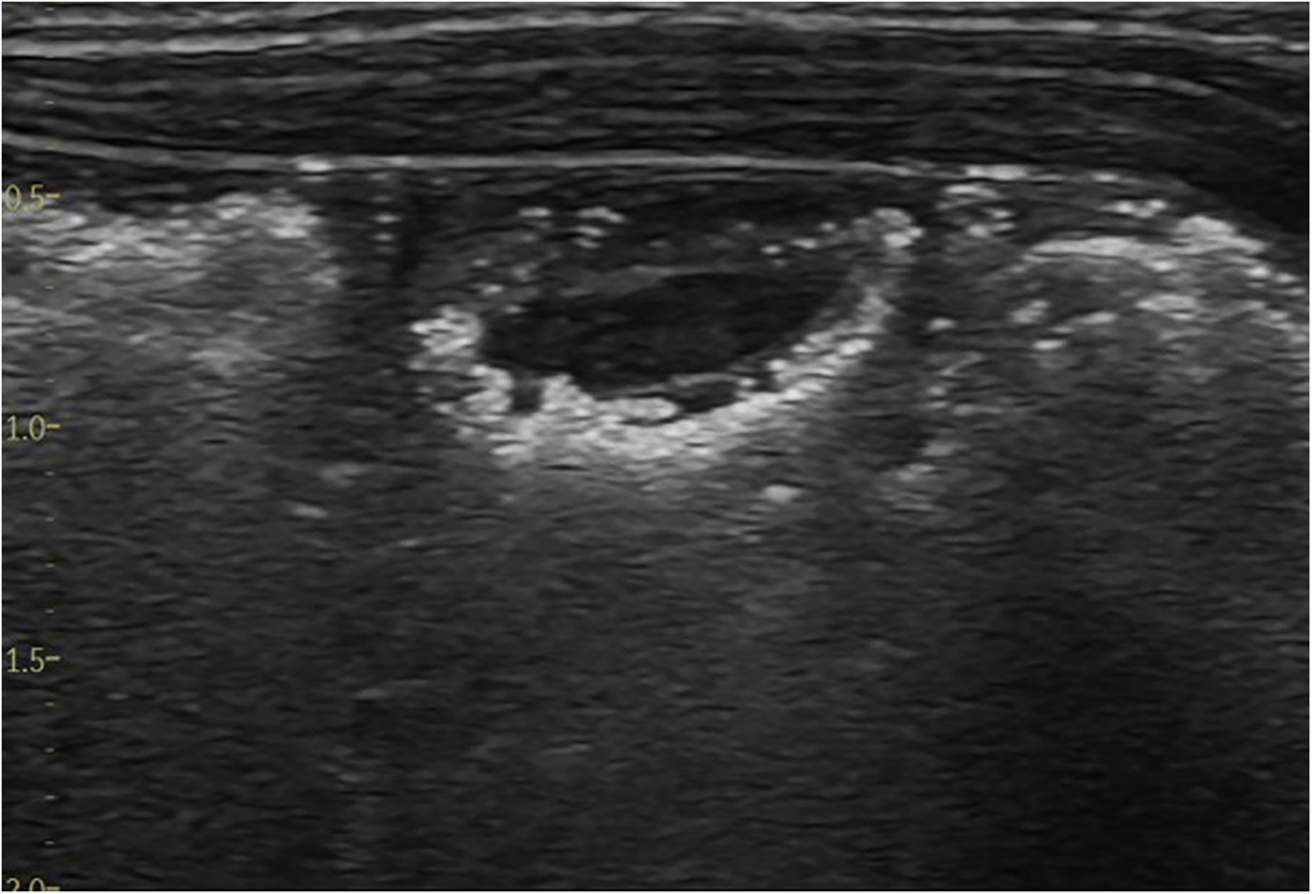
The sonographic appearance of intramural gas trapping—pneumatosis intestinalis, which is seen as white echogenic dots.
